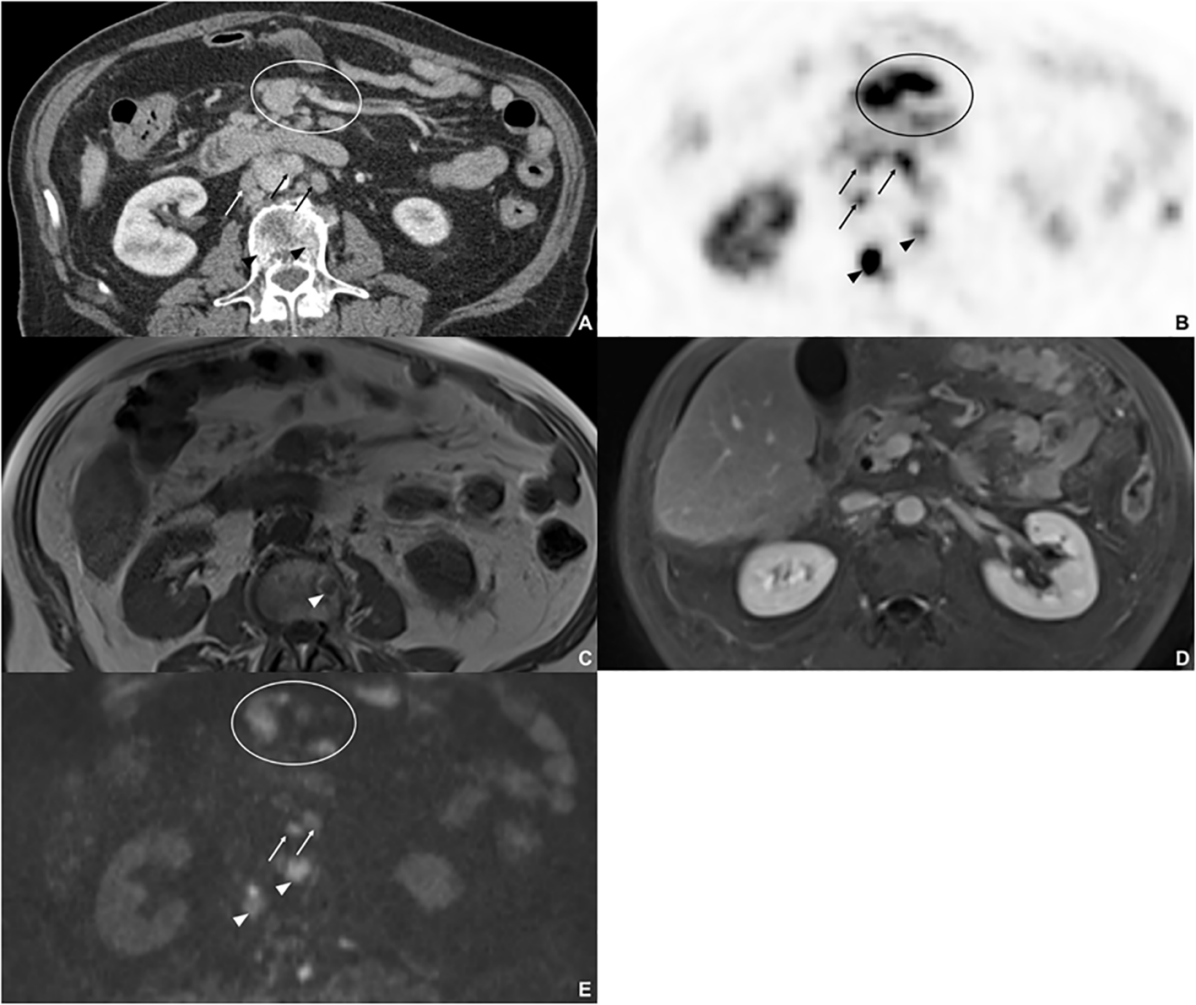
Eighty-five-year-old male with metastatic NET, originating from the gastrointestinal tract. On ^68^Ga-DOTATATE PET/CT (A, B). two sclerotic bone lesions (arrow head), three retroperitoneal lymph node metastases, and mesenterial tumor manifestation with high uptake were detected. On non-contrast T1w image (C), one sclerotic metastasis with low-signal intensity was detected. On the axial contrast-enhanced T1w image (D), no metastases and tumor manifestation were detected, since there was no enhancement of the bone metastases, the lymph node metastases were not rated as metastases due to size and oval shape and mesenterial tumor manifestation was misinterpreted as intestine. On DWI (E), both bone marrow metastases were detected, two of the retroperitoneal lymph node metastases and the mesenteric tumor manifestation.
